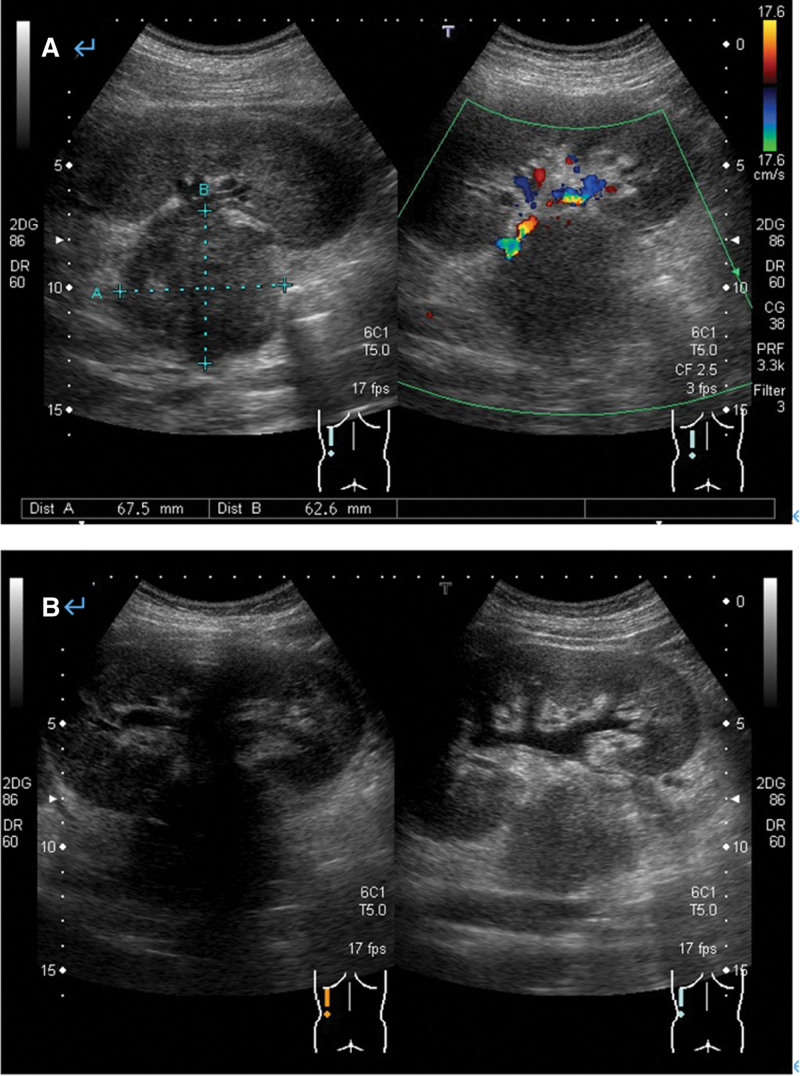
(A, B) Renal ultrasonography. A heterogeneous mass (6.75 × 6.26 cm) compressed the left renal hilum, causing left renal swelling and pelvic dilatation.
